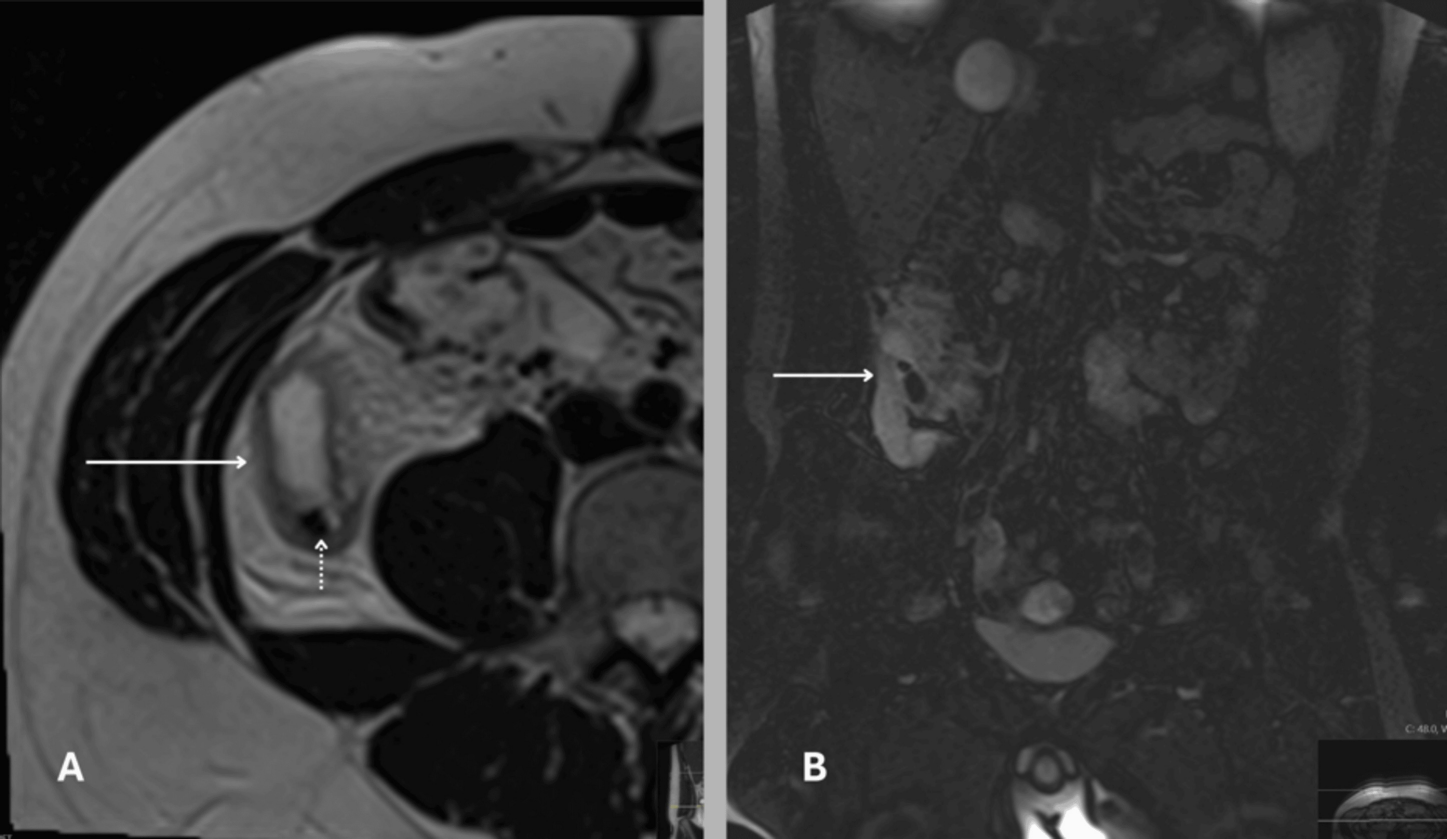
MRI scan of a 23-year-old man from the study showing typical signs of acute appendicitis. A) Axial T2-weighted image showing a distended, thick-walled appendix (arrow), with a surrounding hyperintense area consistent with periappendiceal edema. A small hypointense focus within the lumen suggests an appendicolith (dotted arrow). B) Coronal STIR sequence displaying the appendix (arrow) with similar finding. Image Credit: The figure is original and derived from the data collected in the present study.
